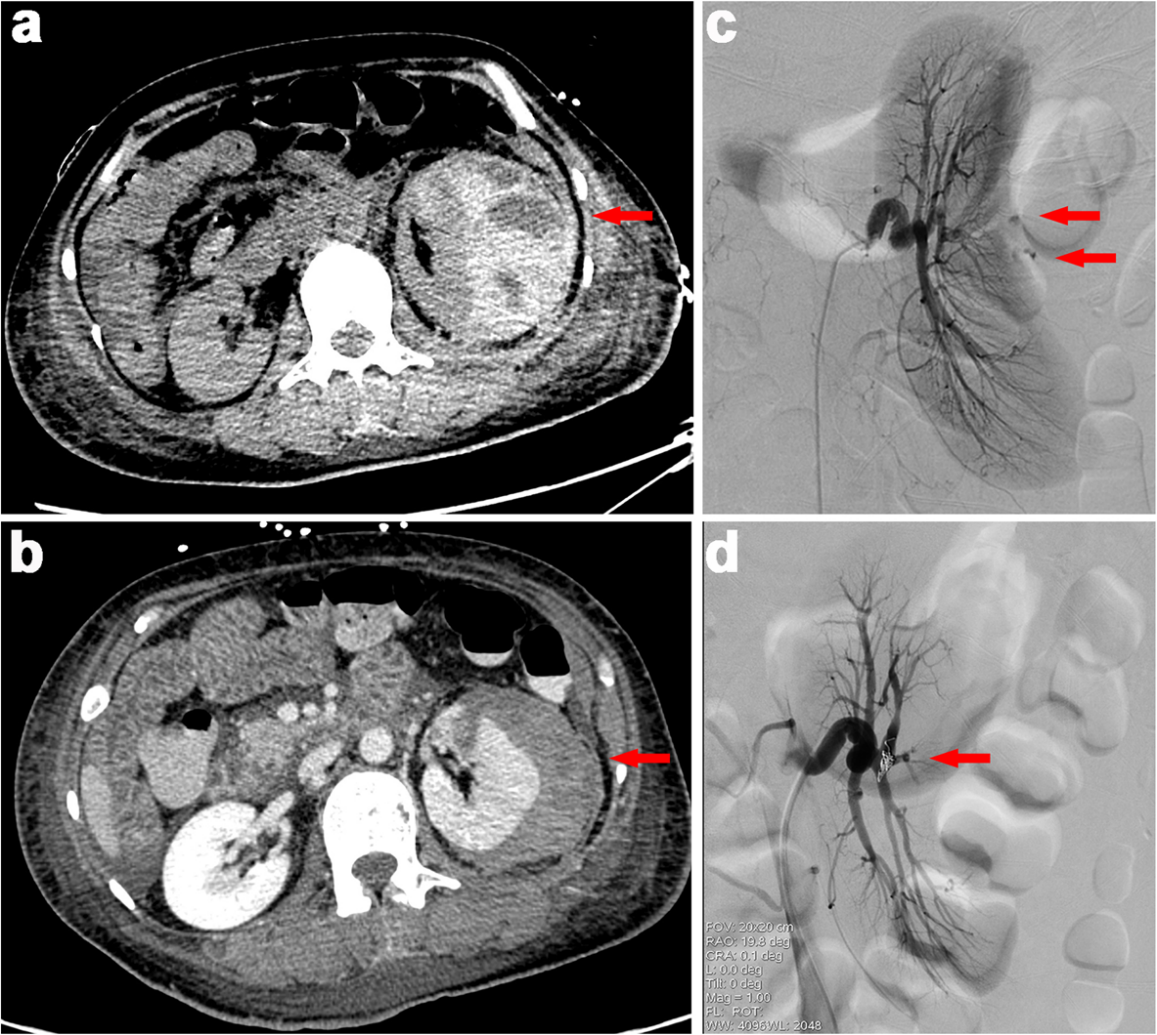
CT and renal artery angiography images: a Abdominopelvic non-contrast CT demonstrated abnormal left kidney contour that was interiorly displaced and externally compressed. A large but limited subcapsular collection with mixed density lateral to the left kidney was seen, with an overall size of 5.8 × 10.1 × 11.7 cm, red arrow; b Contrast-enhanced abdominopelvic CT showed renal parenchymal laceration> 1 cm in depth without collecting system rupture or urinary extravasation. The subcapsular hematoma was shrunk than before with an overall left kidney size of about 4.7 × 8.9 × 11.7 cm, red arrow. In contrast, the right kidney filled uniformly with intravenous dye and showed no obvious sign of injury; c The left kidney was significantly deformed due to suppression. Renal arteriography identified active contrast extravasation from a distant branch of the left renal artery, red arrow; d Post-embolization film showed successful obliteration of bleeding branch, red arrow
